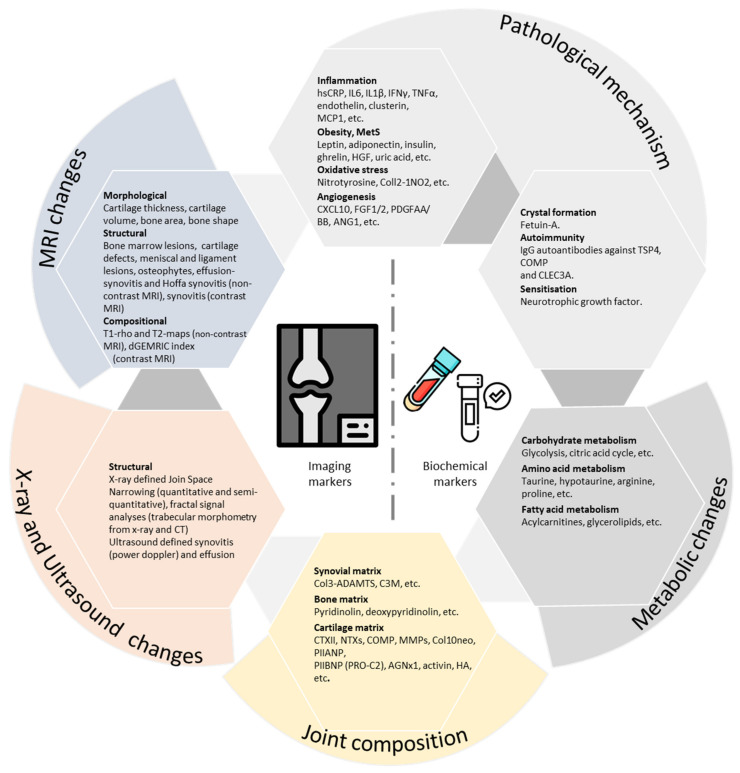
A non-exhaustive representation of osteoarthritis biomarkers. Note: Adapted from: van Spil WE, Szilagyi IA. Osteoarthritis year in review 2019: biomarkers (biochemical markers). Osteoarthritis and cartilage. 1 March 2020;28(3):296-315. Icon designed by Freepik from www.flaticon.com. Access date: 24 June 2021. AGNx1: ADAMTS-degraded aggrecan; ANG: angiopoietin; BML: bone marrow lesion; C3M: collagen type III degraded by matrix metalloproteinase; CLEC3A: C-type lectin domain family 3 member A; Coll2-1NO2: nitrated epitope of the a-helical region of type II collagen; COL3-ADAMTS: collagen type III cleavage product derived from ADAMTS; Col10neo: collagen typeX neo-epitope; COMP: cartilage oligomeric matrix protein; CTXII: C-terminal cross-linked telopeptide of collagen type II; CXCL10: C-X-C motif chemokine 10; dGEMRIC: delayed gadolinium-enhanced MRI of cartilage; FGF: fibroblast growth factor; HGF: hepatic growth factor, hsCRP: high sensitivity C-reactive protein; IL: interleukin; IFN: interferon; MCP: monocyte chemoattractant protein; MMPs: matrix metalloproteinases; NTX: N-telopeptide crosslinks; PDGF: platelet-derived growth factor; PIIANP, PIIBNP: N-terminal propeptide of type II collagen, splice variants IIA and IIB, respectively; TNF: tumour necrosis factor; TSP: thrombospondin; US: ultrasound; MRI: magnetic resonance imaging.
